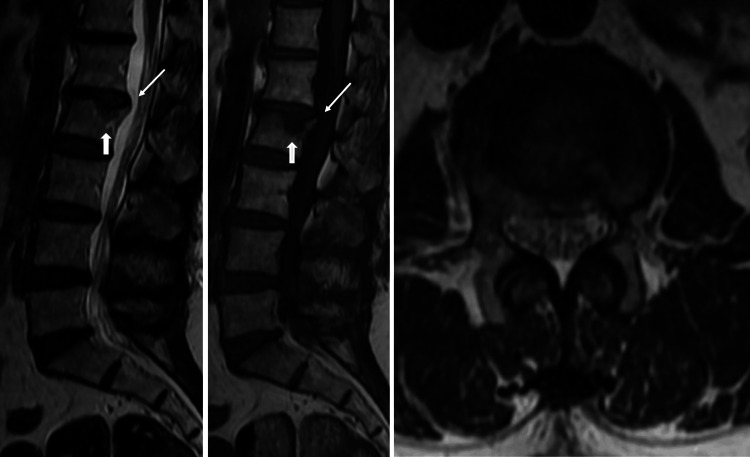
Sagittal T2 (A) and T1 (B), and axial T2-weighted (C) images show a subchondral geode (thick arrow) in the superior plateau of the L2 vertebra, which associates a small disc herniation and posterior limbus of the superior vertebral corner (thin arrow). The image credits belong to the authors.
